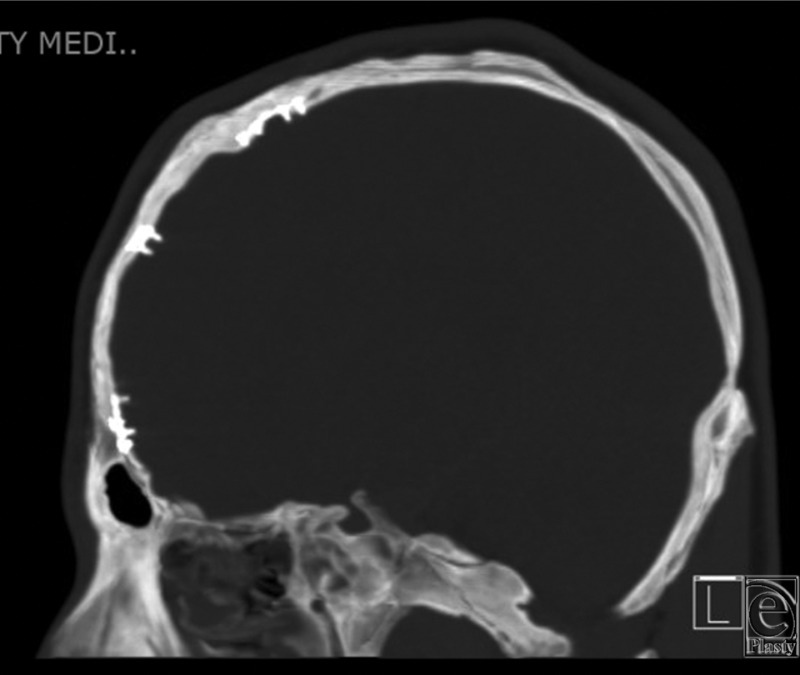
Sagittal computed tomographic scan of intracranial extension of hardware.
